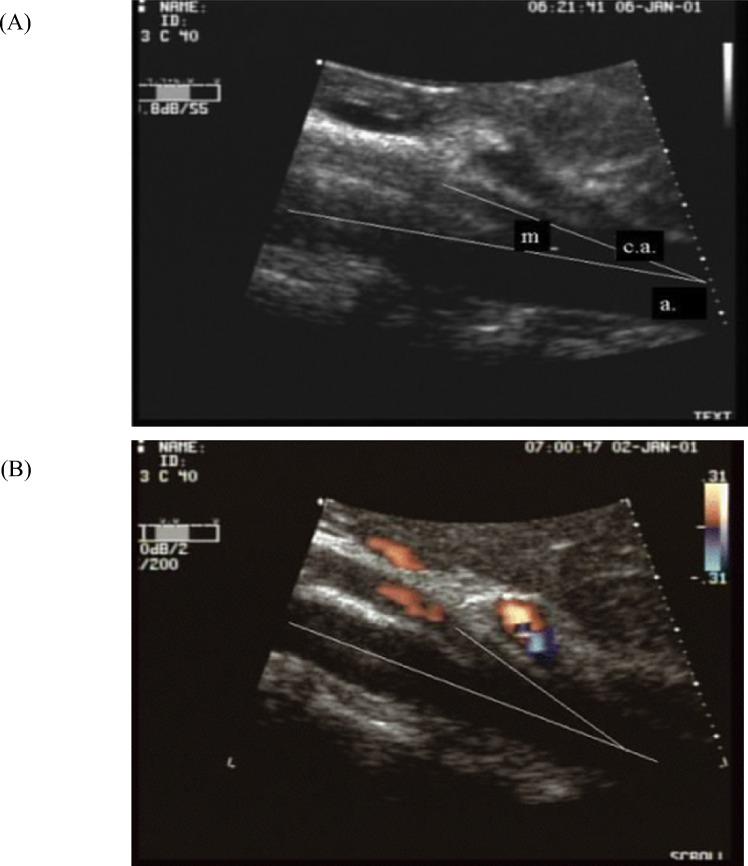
Superior mesenteric artery syndrome. A Ultrasound of supine patient; measuring aortomesenteric artery at 14 degrees. B Echo Color Doppler of patient lying down: measuring aortomesenteric artery at 21 degrees
